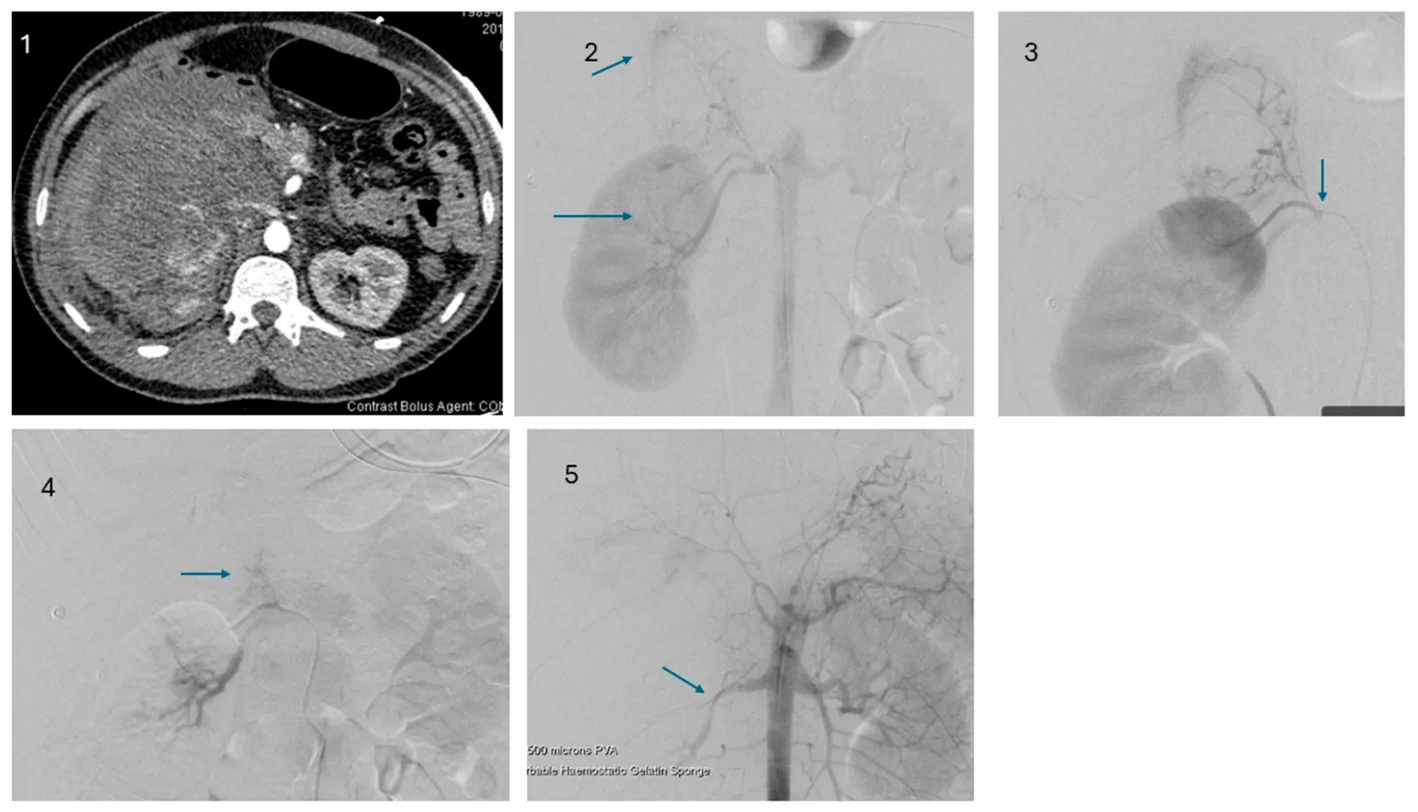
Case 8: 1. CT with IV contrast: right hematoma rising from rupture, right adrenal mass with active stabilization of contrast on the arterial phase. 2–5. Angio renal artery: 2 = right adrenal with abnormal arterial supply to the tumor from right adrenal artery. 2 = right renal artery. 3 = selective right adrenal angiogram. 4 = embolized gel foam right adrenal artery. 5 = gelfoam is spilled into the right renal artery with partial occlusion of right renal artery.
